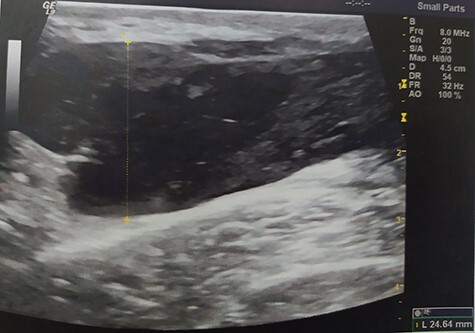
Pelvic ultrasound indicating an acute hematoma into the fatty tissue of the right iliac fossa.
